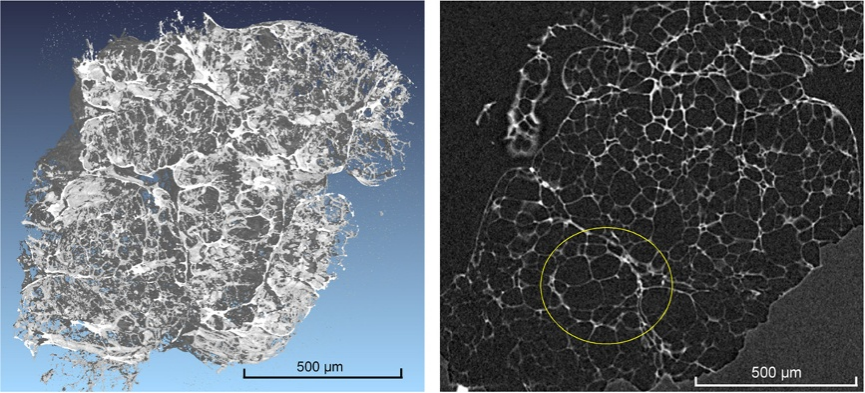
Microstructural investigation of expanded perlite by CT (left pseudo 3D image, right 2D cross-section). The circle shows that small pores with thinner walls are included in spherical structures with somewhat larger walls.
